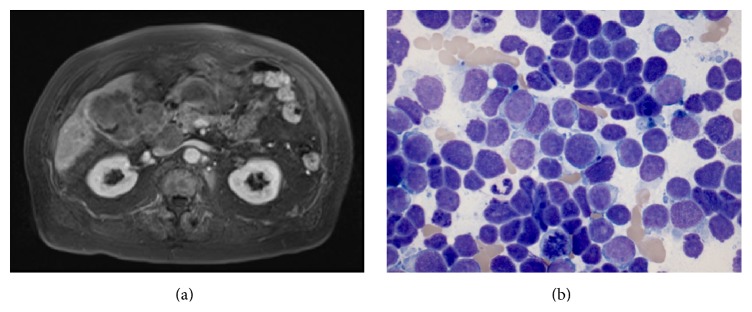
Patient case MRI and FNA of liver lesion. (a) MRI of the abdomen showed a bulky gallbladder tumor measuring 5.6 cm with direct extension into the liver, innumerable hepatic and periportal nodal metastases which exerted mass effect on the cystic duct, and peripancreatic and right diaphragmatic nodal involvement. (b) Ultrasound-guided biopsy of a metastatic liver lesion shows small and fragile epithelioid cells with little cytoplasm and fine chromatin. Abundant apoptotic bodies and mitotic figures were seen consistent with small cell carcinoma.
